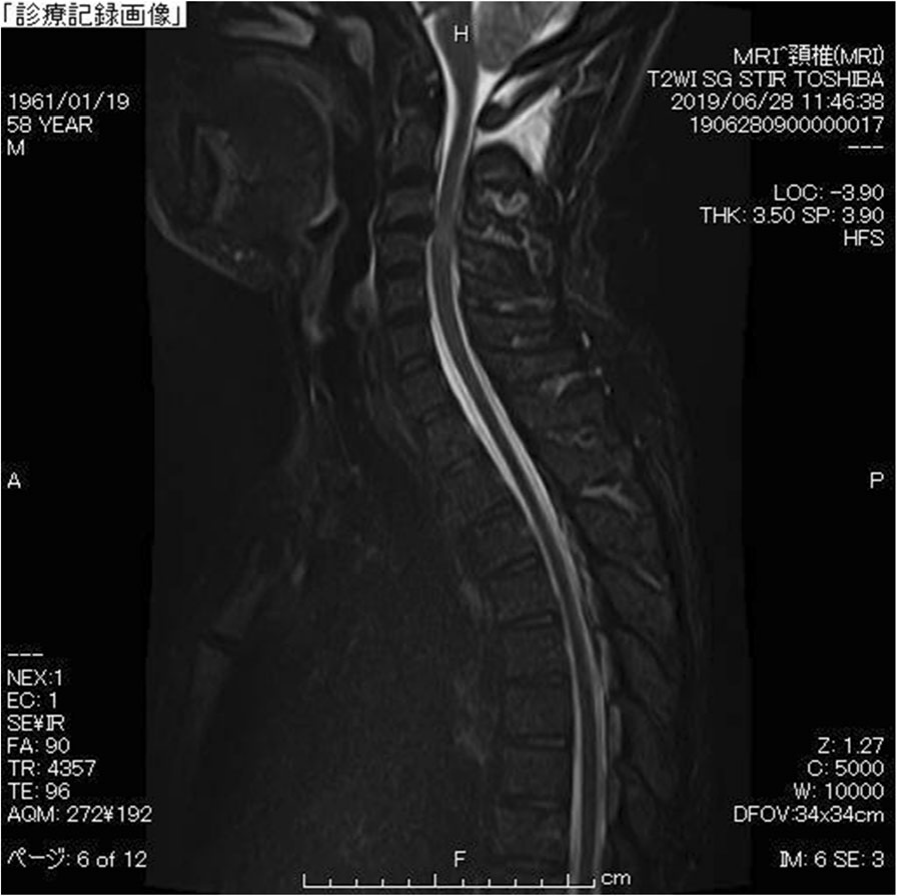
C1-C2 sign. A saggital view of T2-weighted fat suppression image of magnetic resonance imaging (MRI) in the cervical level revealed fluid collection in the retrospinal region at C1-C2 level. Additionally, a spinal canal stenosis at C3/4 level was also demonstrated
